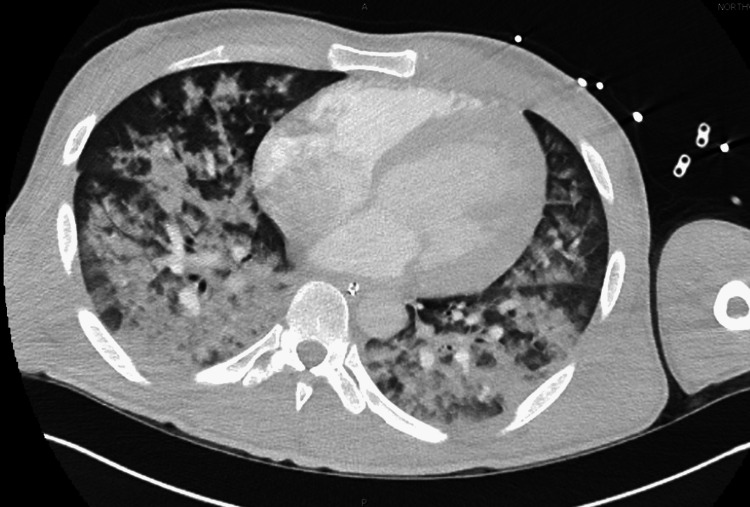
Computed tomography with angiography of the chest showing diffuse multifocal opacities
